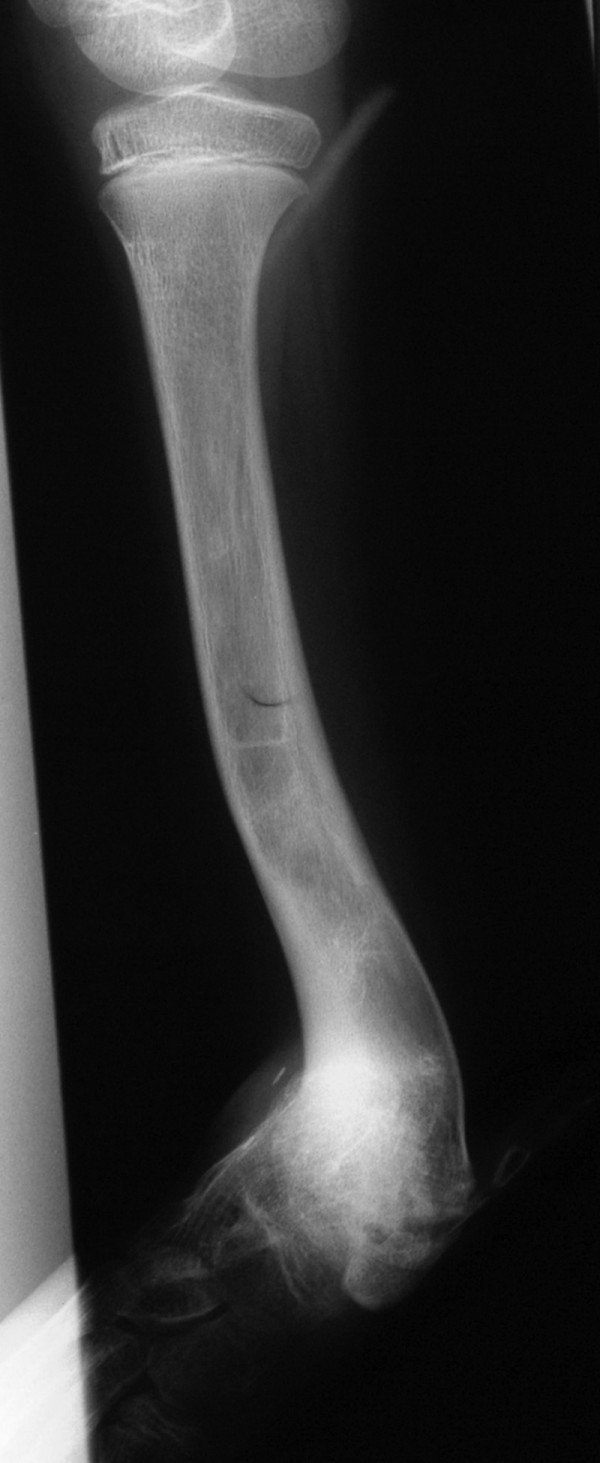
Radiographic presentation of the same patient two years after lengthening procedure. Severe valgus deformity of 85° of the distal tibia in the age of nine y.o., and two years after completing a lengthening procedure of the tibia. Despite multiple efforts to correct axial malignment during lengthening this was not gained and a severe valgus deformity of the ankle with a prominent antero-medial bowing of the tibia were obvious. It is believed that a severe dysplasia of the lateral distal epiphysis of the tibia is mainly responsible for this resisting to treatment deformity.
